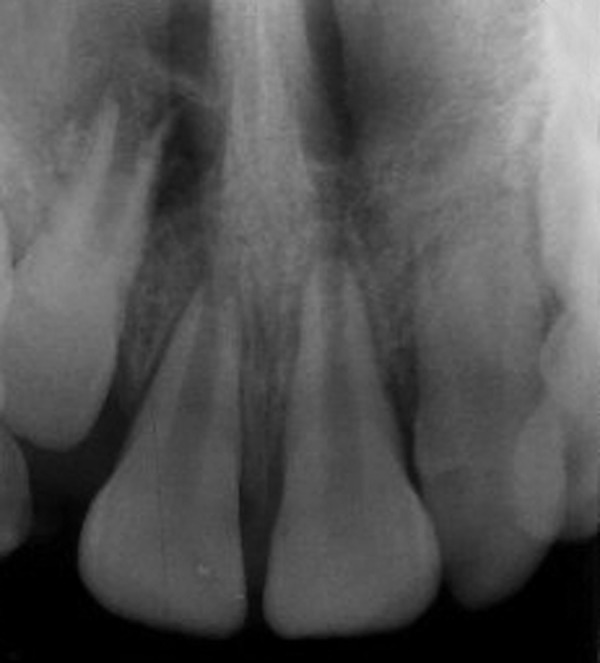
Preoperative intraoral periapical radiograph (case 5)
